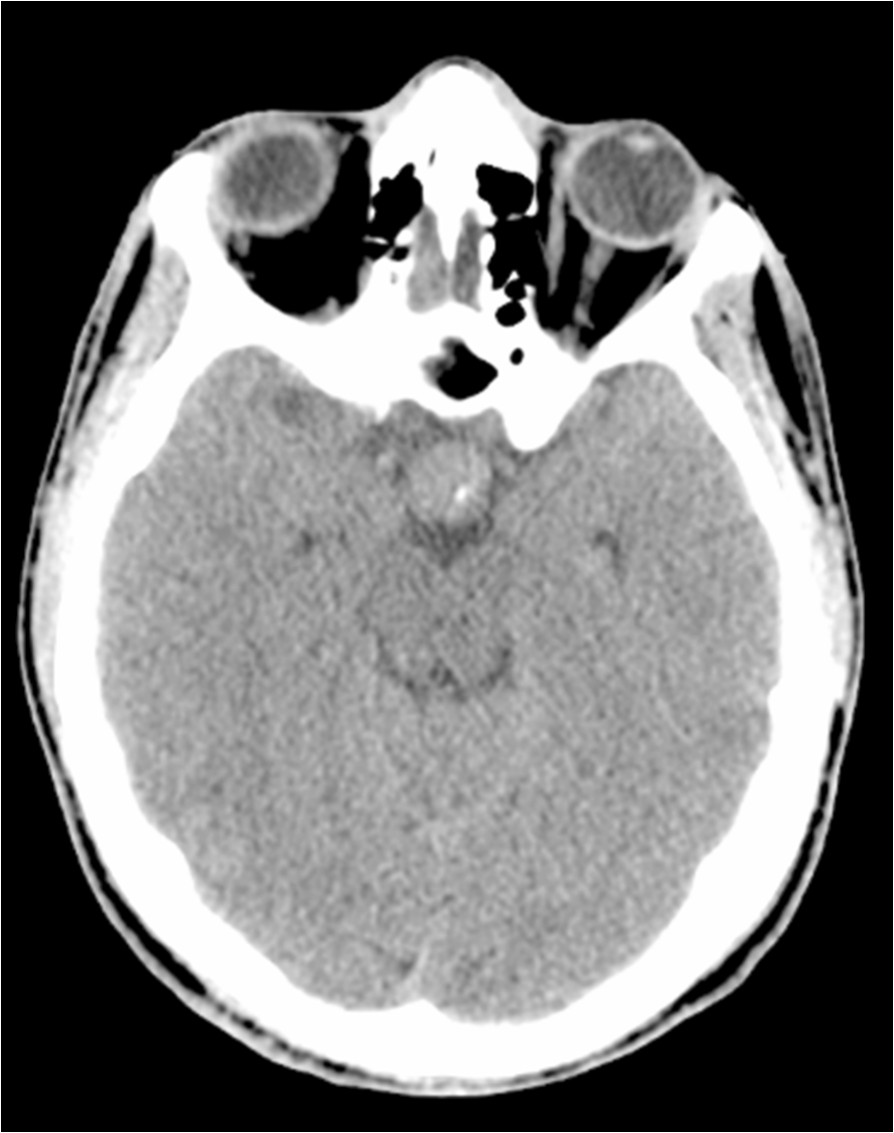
Cross-sectional computed tomography scan showing a pituitary mass.
